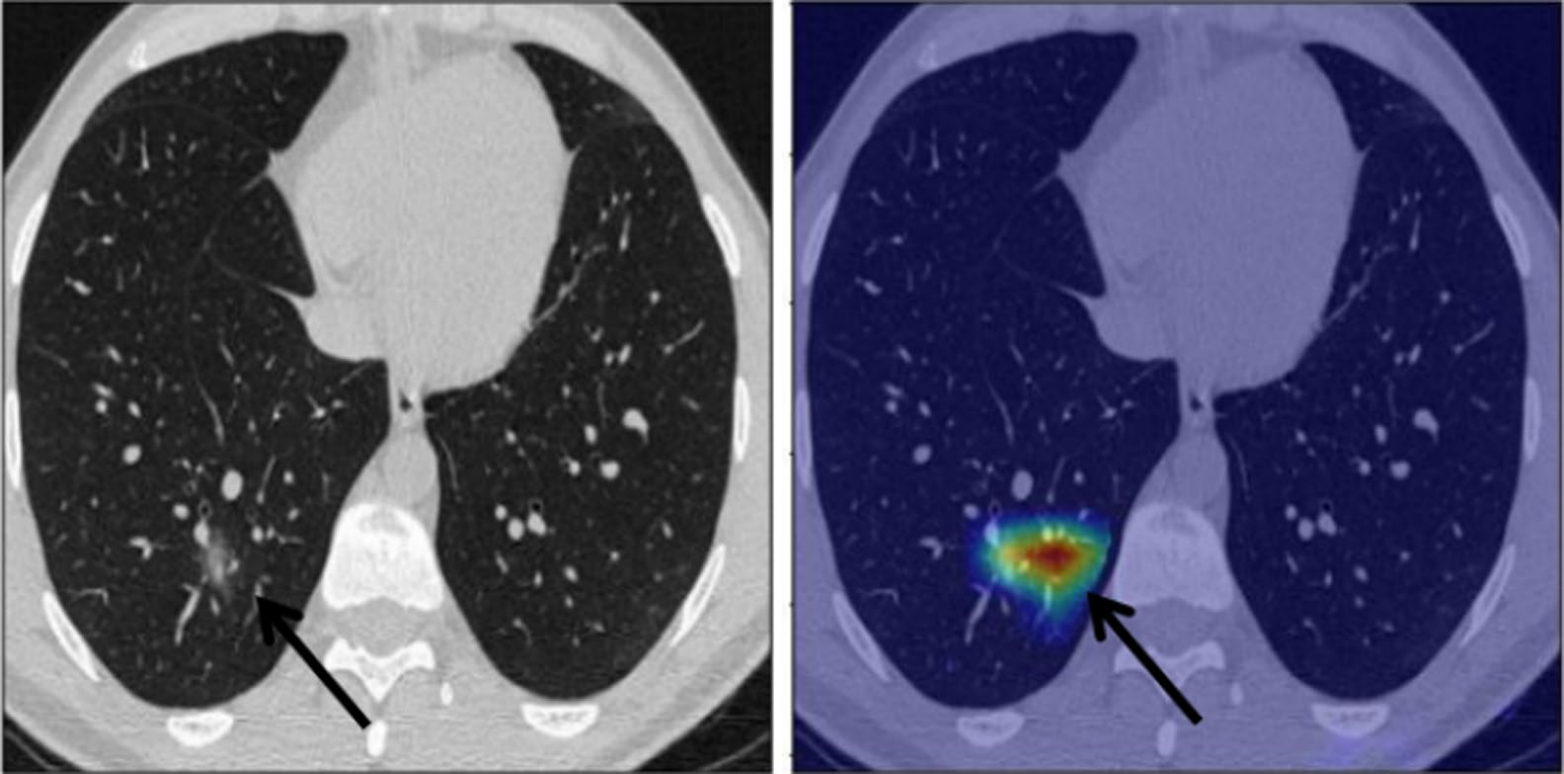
A 34-year-old male patient presented with COVID-19-positive PCR test and suffering from cough and chest pain. CT chest was performed with color-coded images showing left lower patchy of ground glass opacity which was better visualized in color-coded image
